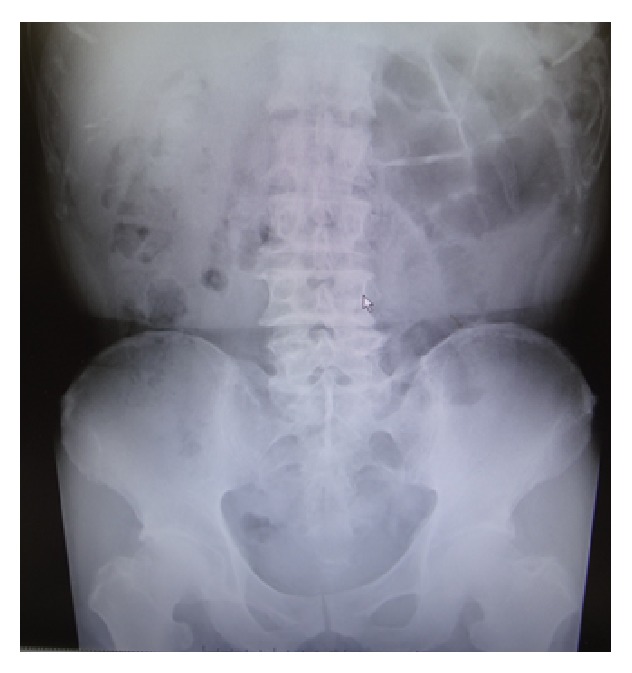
A radiograph shows the dilated small bowel in the upper quadrant.
